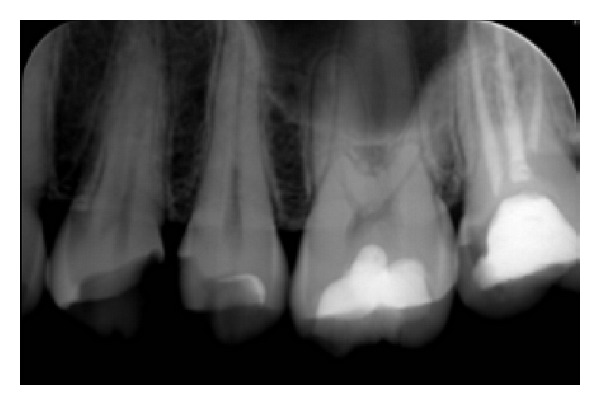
Initial radiography showing proximal cavity on tooth number 27.
